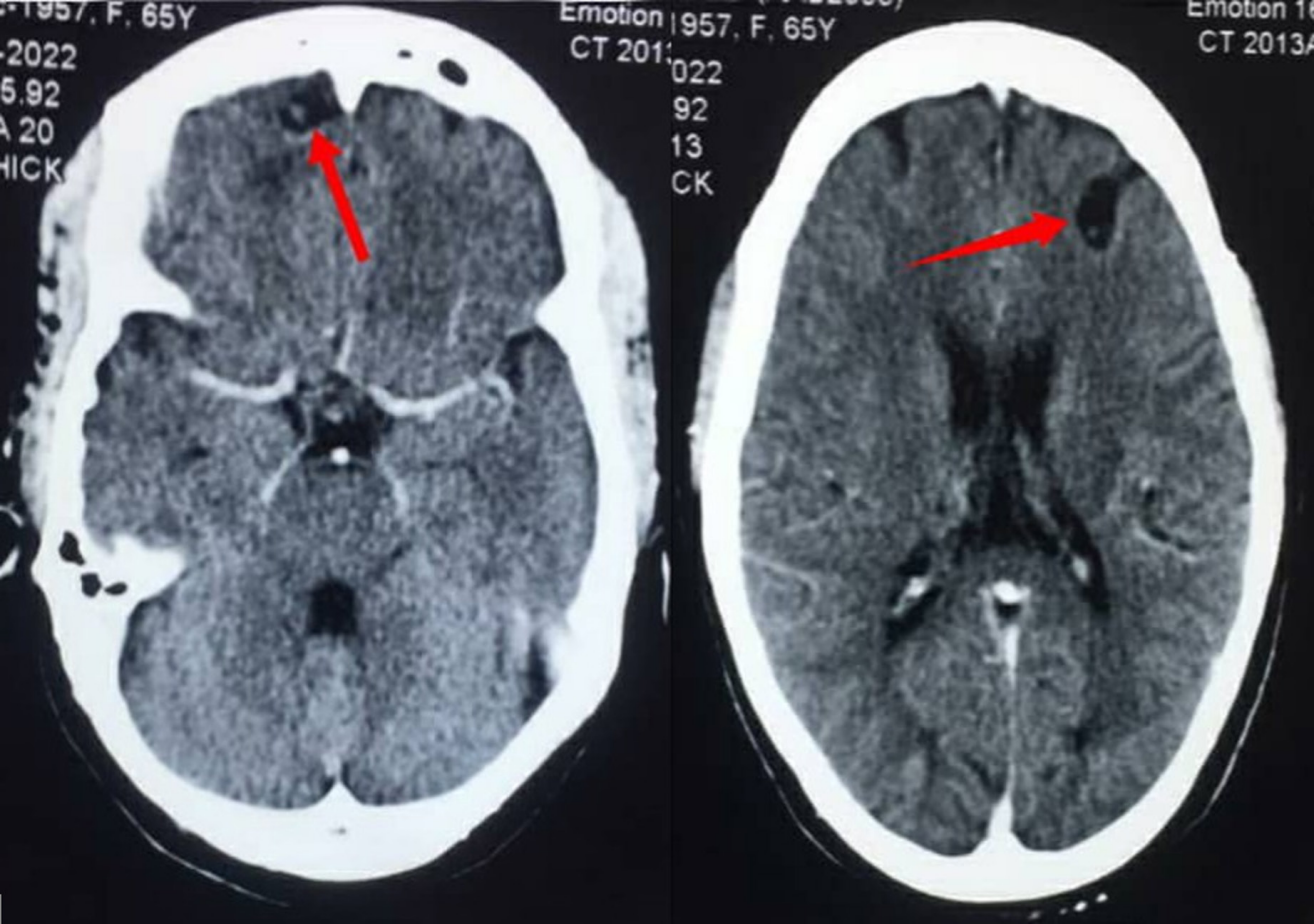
Contrast enhanced axial computed tomography (CT) scan images of the brain showing cystic lesions with eccentric hyperdense foci (cysts with scolices) in both frontal lobes.
